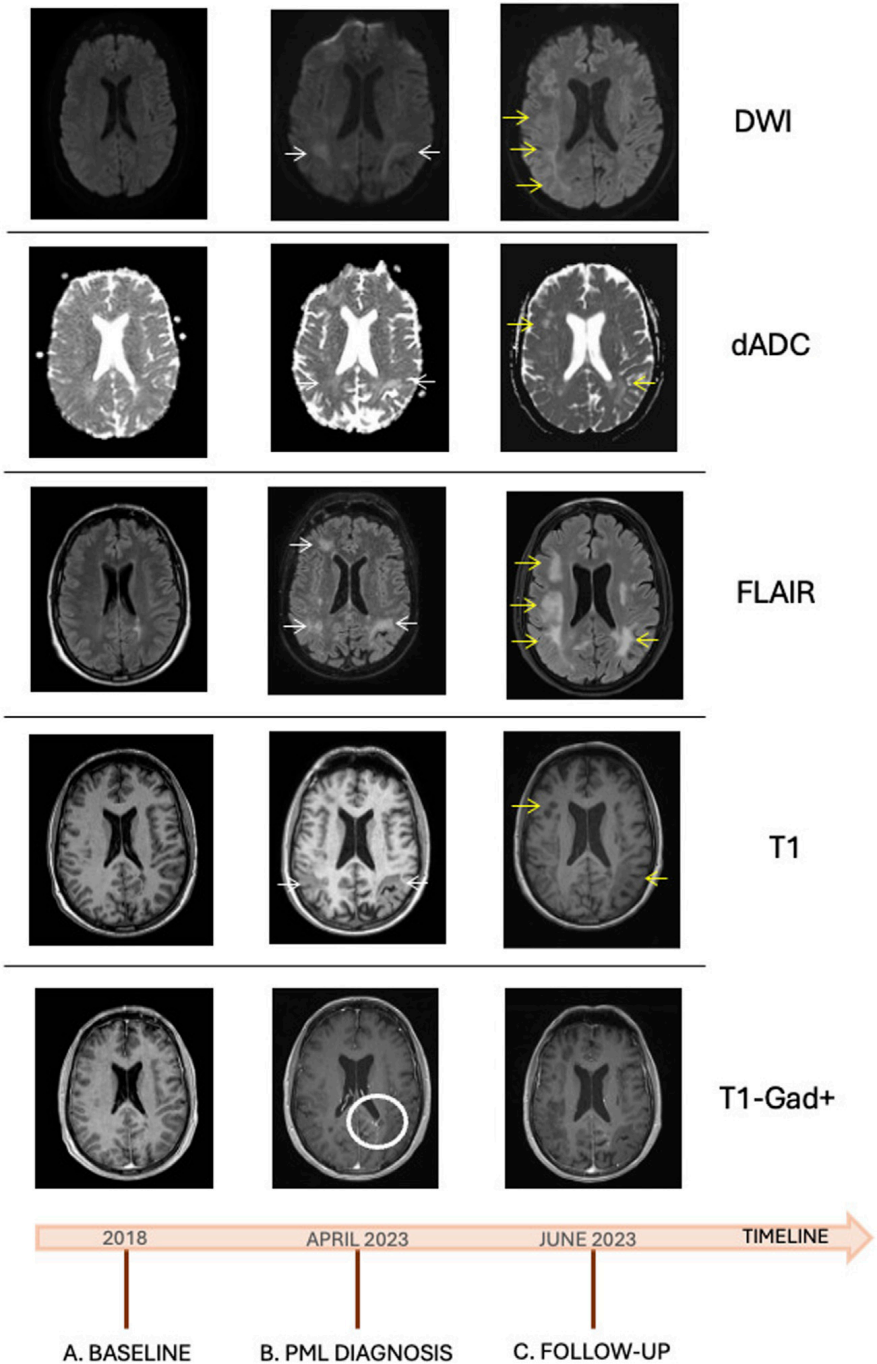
Neuroimaging study during follow up: (A) baseline MRI, 6 months before OCR treatment onset, observing hyperintense T2/FLAIR lesions with no contrast enhancement or diffusion restriction; (B) MRI performed upon PML diagnosis, observing subcortical lesions and lesions in both parietal and occipital lobes, T1 hypointense and FLAIR hyperintense (white arrows), with no expansive effect, contrast enhancement, or diffusion restriction. Contrast enhancement in the left parieto-temporal region related to a venous development anomaly, previously known (white circle) (C) Follow-up MRI after symptom worsening, observing an expansion of PML lesions (yellow arrows), with no contrast enhancement or diffusion restriction. dADC, Apparent diffusion coefficient ADC map; DWI, Diffusion-weighed imaging; FLAIR, fluid-attenuated inversion recovery; PML, progressive multifocal leukoencephalopathy; OCR, ocrelizumab; MRI, magnetic resonance imaging; T1-Gad+, T1 sequence with gadolinium.
